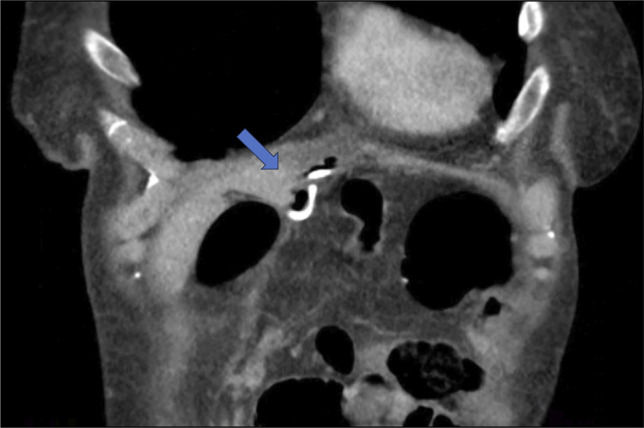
Computed tomography of the abdomen revealed migration of the stent into a perigastric sinus tract.
